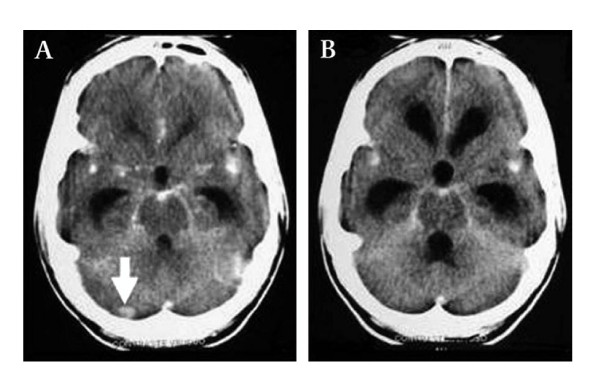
CT scan of head showing basal meningeal enhancement on contrast study. Arrow points to a tuberculoma.
